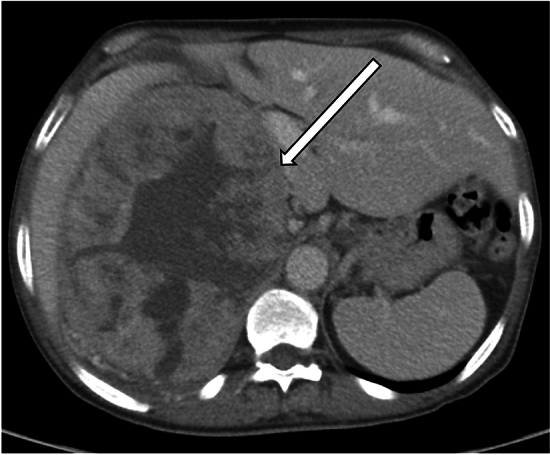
A representative case of ACC in a 64-year-old man with abdominal pain. The axial CT image of the right adrenal gland demonstrated a large necrotic mass (arrow) with associated mass effect on the adjacent viscera. Retroperitoneal lymphadenopathy was present as well (not shown). Pathology was consistent with primary adrenocortical carcinoma, with invasion into the periadrenal/renal adipose tissue
